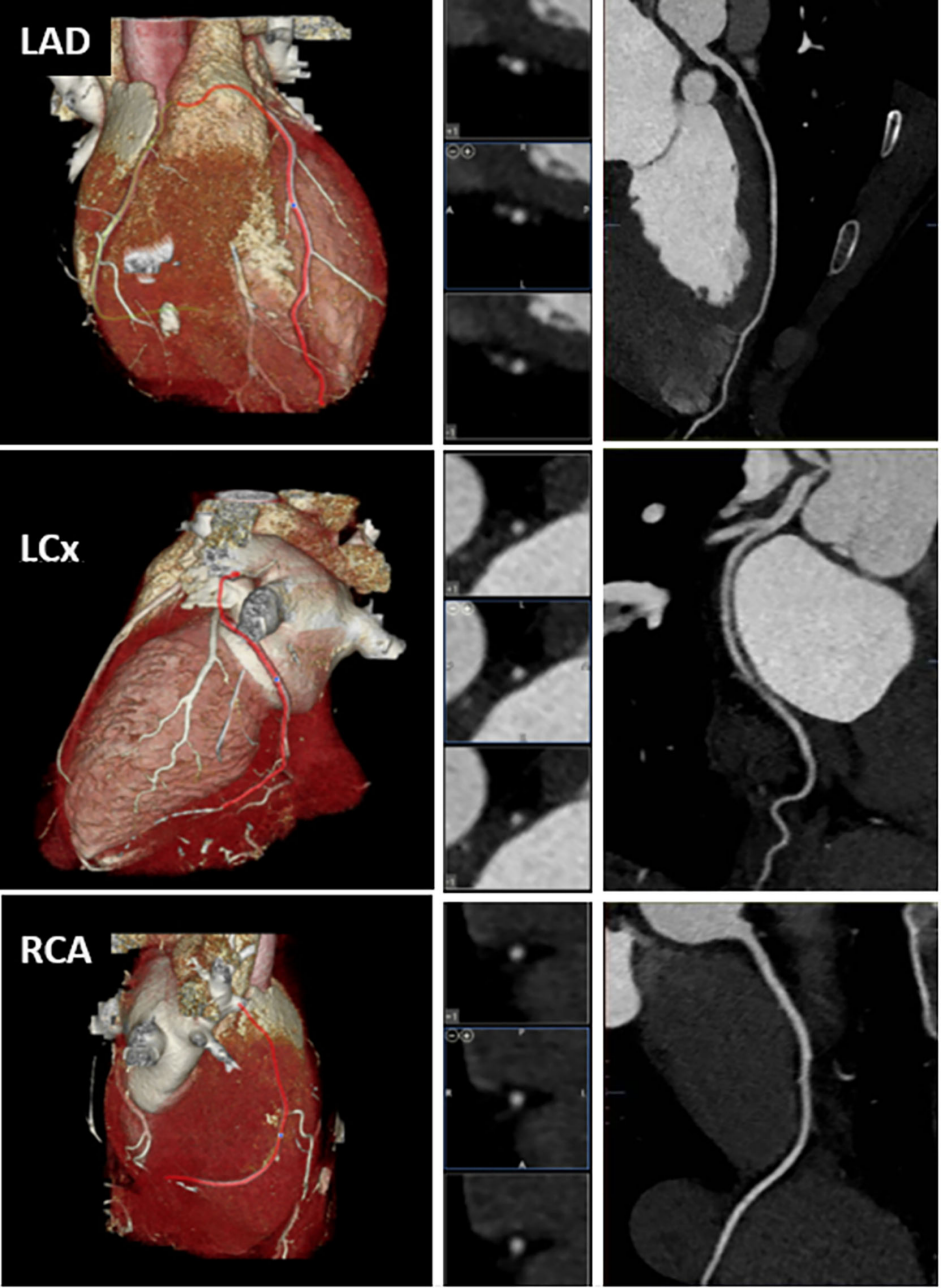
Computed tomographic angiogram demonstrating no coronary artery disease. Left column: Volume-rendered reformations. Middle column: Multiplanar reformation cross-sectional views. Right column: Curved multiplanar reformations. LAD, Left anterior descending artery; LCx, Left circumflex artery; RCA, Right coronary artery.
